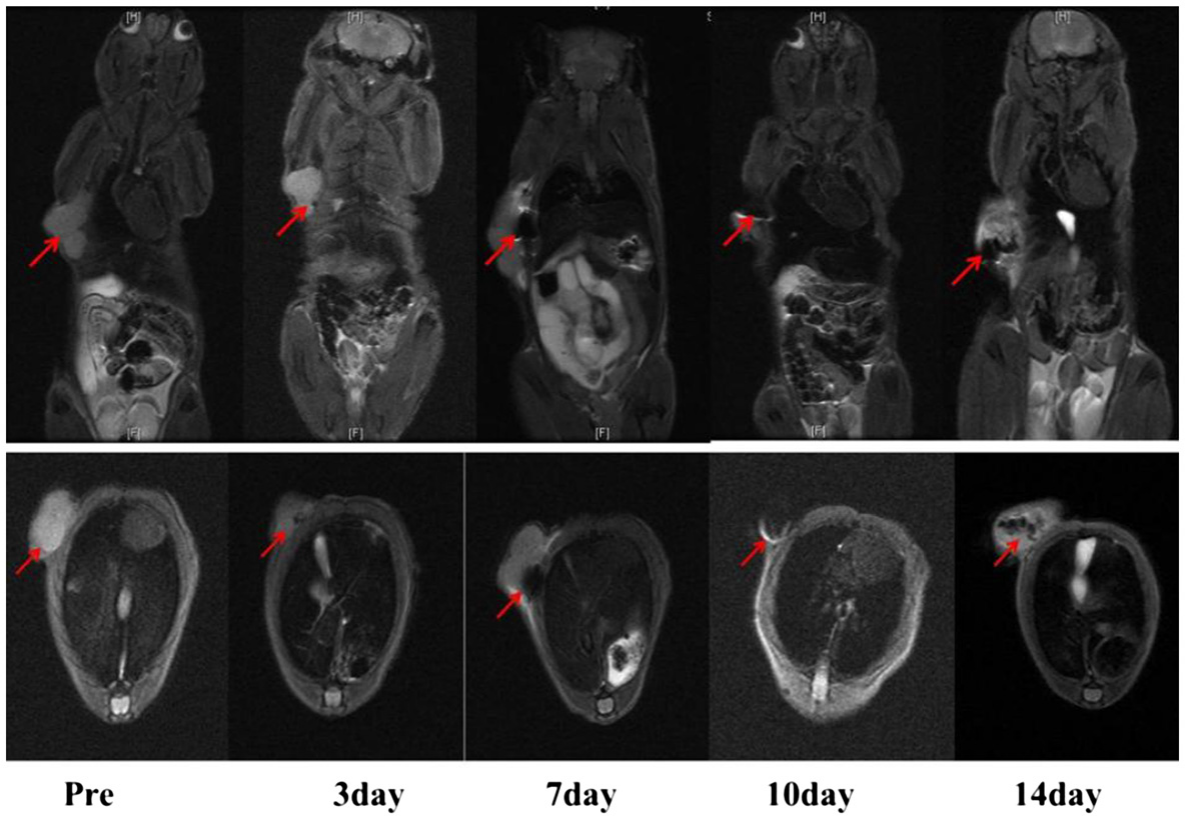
MR imaging of FMNP-labeled MSCs targeting gastric cancer cells. The coronal and transected images of MRI show that MSCs firstly presented in the tumor tissue at 7 days post-injection and then gradually accumulated in the tumor tissue and reached peak value at 14 days post-injection.
